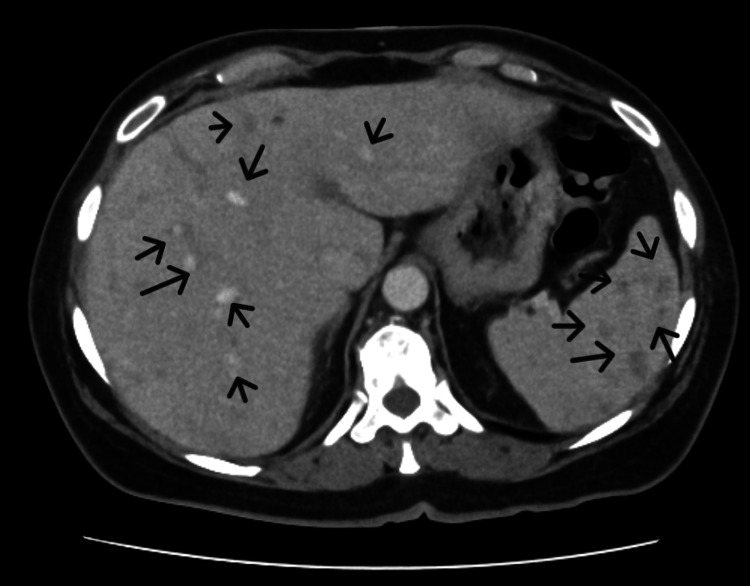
CT abdomen and pelvis revealed enlarged liver, heterogenous parenchyma with ill-defined patchy low density lesions Enlarged spleen with several small focal lesions.
